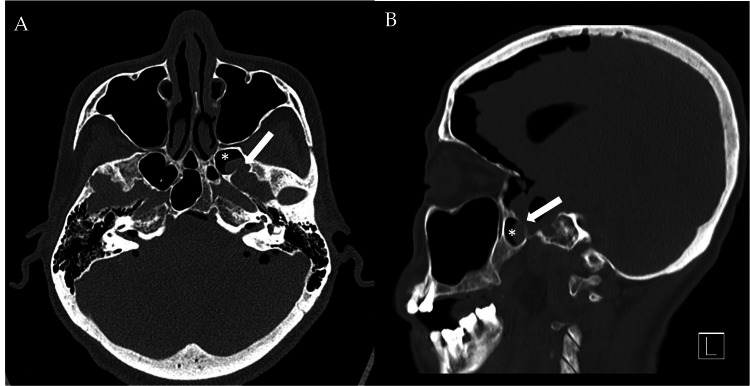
Non-contrast CT skull base in axial (3A) and sagittal (3B) showing focal bony dehiscence (white box arrow) in the posterolateral wall of the left sphenoid sinus (white asterisk) with density protruding within possibly representing encephalocele.
